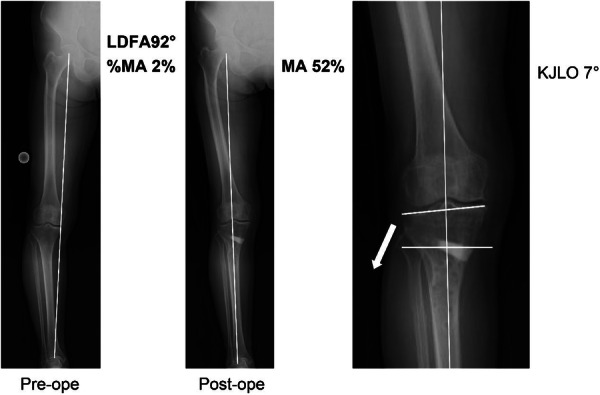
Radiographs demonstrating alignment correction pre‐ and post‐op from a representative case. Knee joint line obliquity (KJLO), lateral distal femoral angle (LDFA), mechanical axis (MA).
